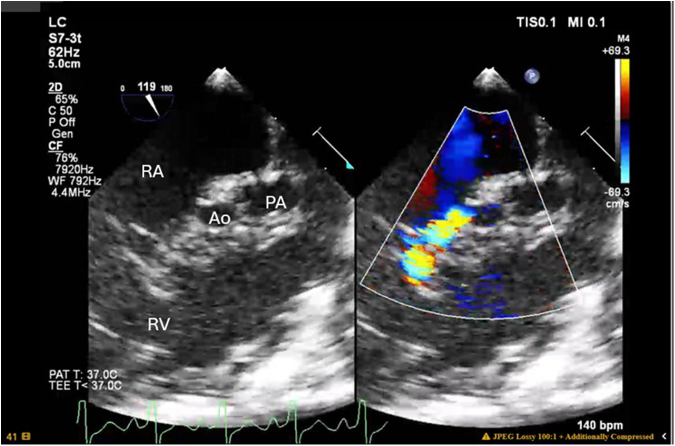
Intraoperative transesophageal echocardiogram demonstrating shunt flow from the aorta to right atrium/right ventricle. *RA*, Right atrium; *Ao*, aorta; *PA*, pulmonary artery; *RV*, right ventricle.
